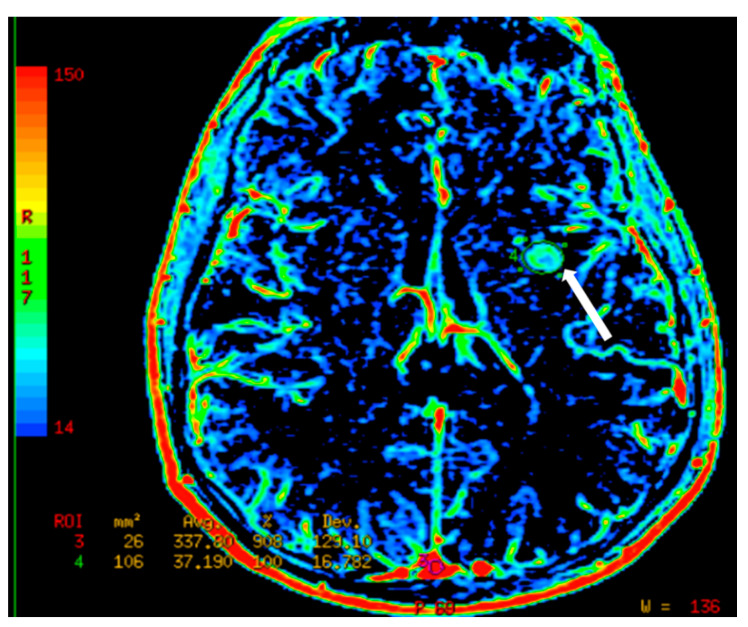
Axial dynamic contrast-enhanced (DCE) MRI of the brain with a relative cerebral cerebral blood volume (rCBV) map, which shows relatively increased flow dynamics in the left basal ganglia lesion (arrow).
